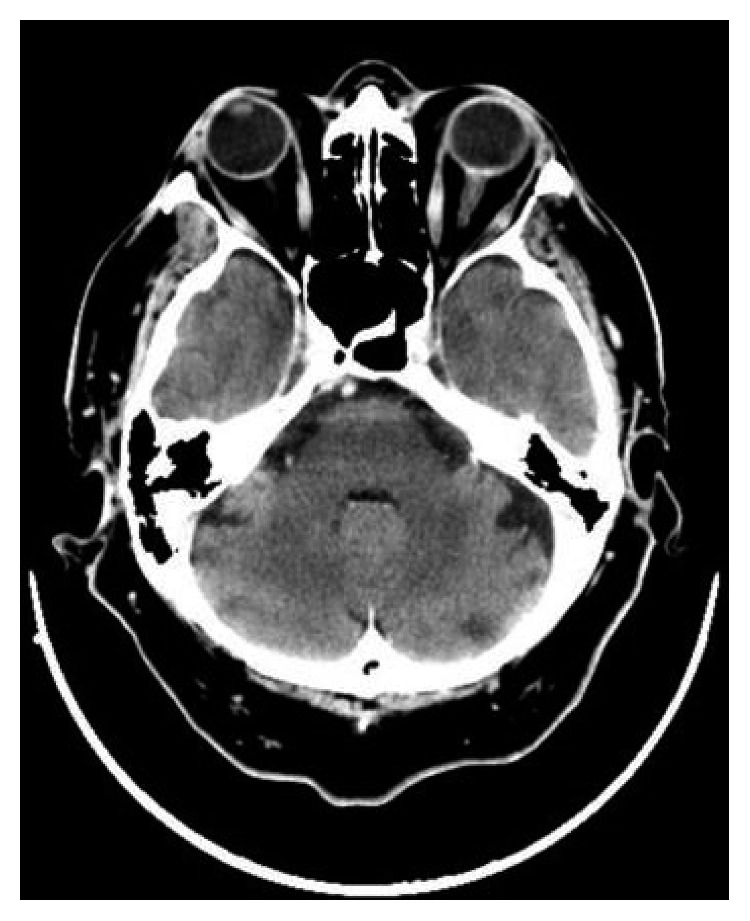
The CT scan performed after the end of the salvage therapy showed complete remission of the disease.
